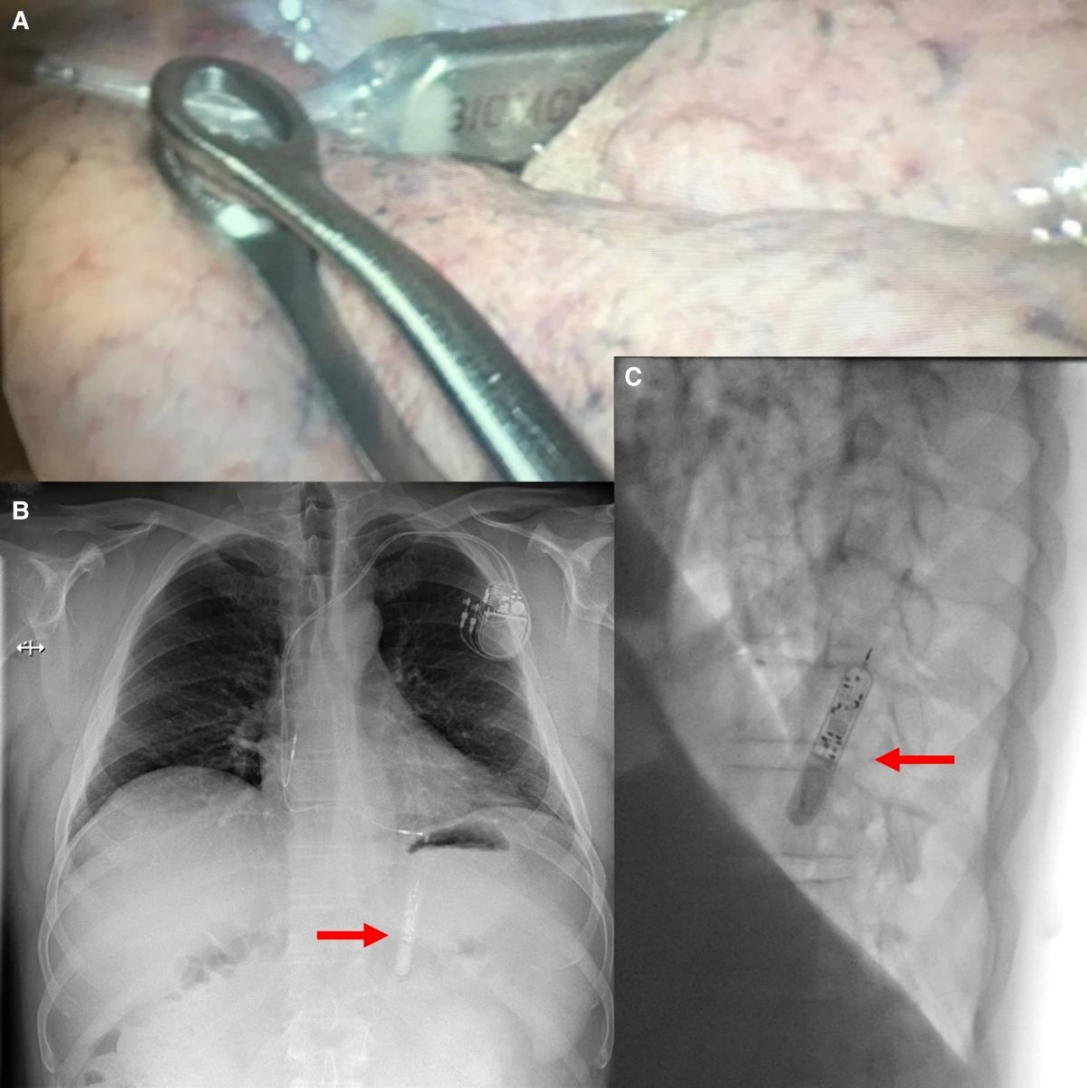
(*A*) Thoracoscopy with intrapleural loop monitor; (*B*) chest X-ray face (first post-implantation imaging); (*C*) chest X-ray profile (Pre-operative imaging).
